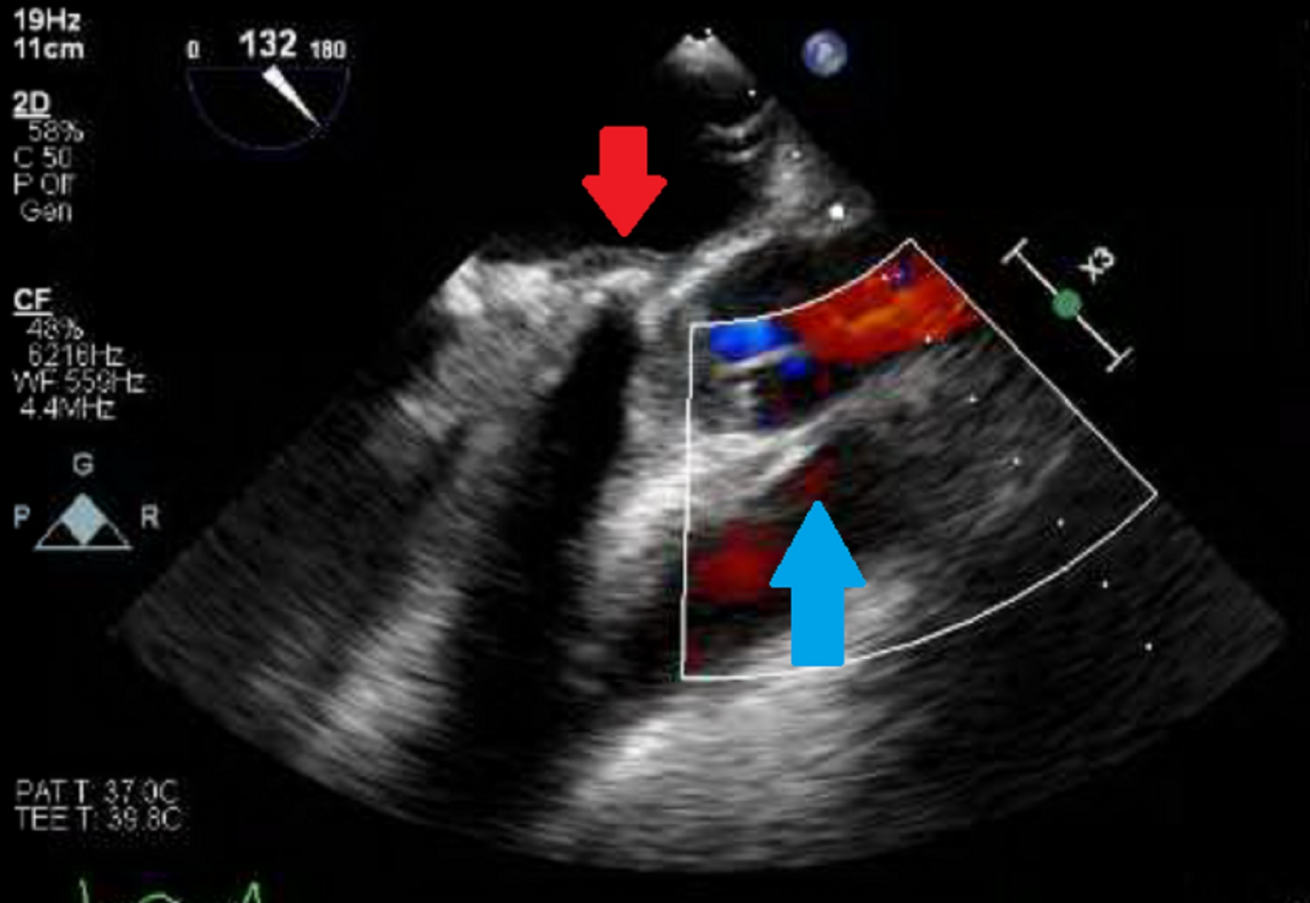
Transesophageal echocardiogram with severe stenosis and vegetation on prosthetic mitral valve (red arrow) and severe aortic stenosis (blue arrow)
